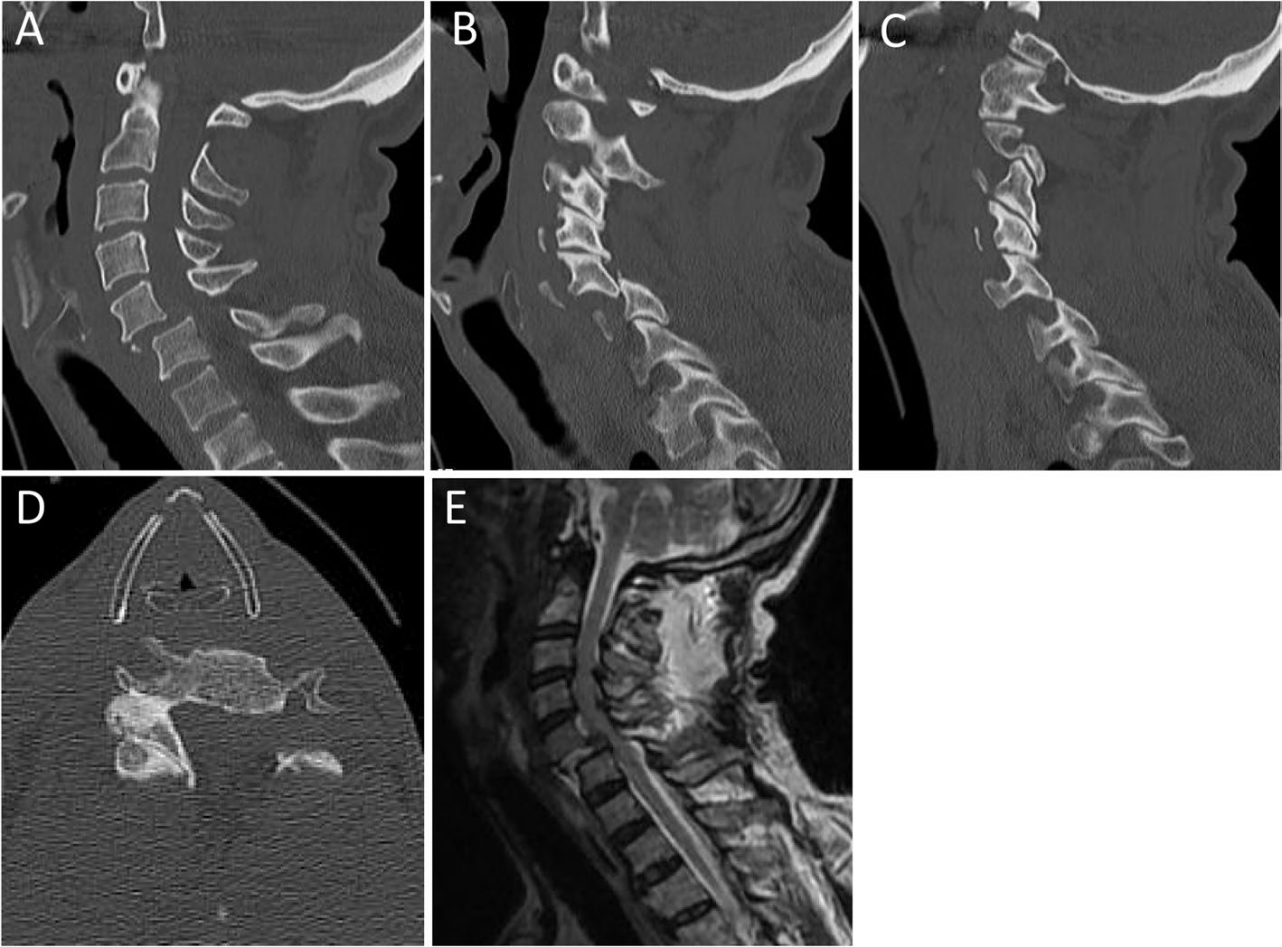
Preoperative CT scan showing a cervical dislocation at the C5/6 level: (A) central part of the sagittal reconstruction view, (B) right facet view, (C) left facet view, and (D) axial image. (E) Preoperative MRI showing severe spinal cord compression at the C5/6 level by the disc and the posterior supporting structure
